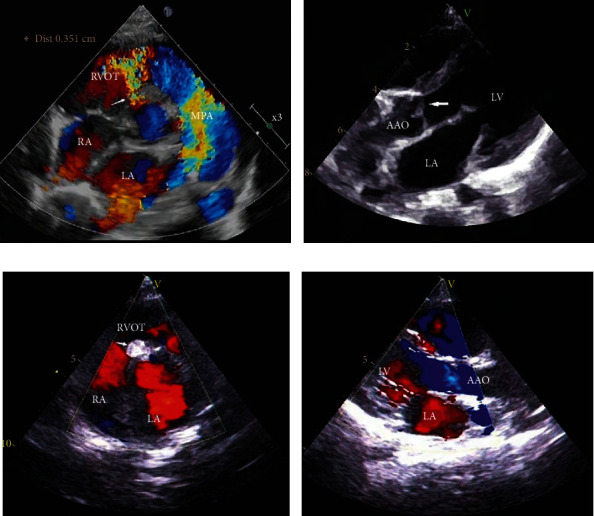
(a) Transthoracic echocardiography (TTE) showing the location of perimembranous ventricular septal defect and color Doppler image demonstrating a left to right jet through the defect (white arrows). (b) Moderate AVP is evident (white arrows). (c) TTE demonstrating optimal occluder position and absence of residual shunt after release of the occluder (white arrows). (d) After successfully implanting VSD occluder, parasternal long-axis TTE confirms no AR (LA = left atrium; RA = right atrium; RVOT = right ventricular outflow tract; MPA = main pulmonary artery; LV = left ventricle; AVP = aortic valve prolapse; AR = aortic regurgitation; VSD = ventricular septal defect; AAO = ascending aorta).
